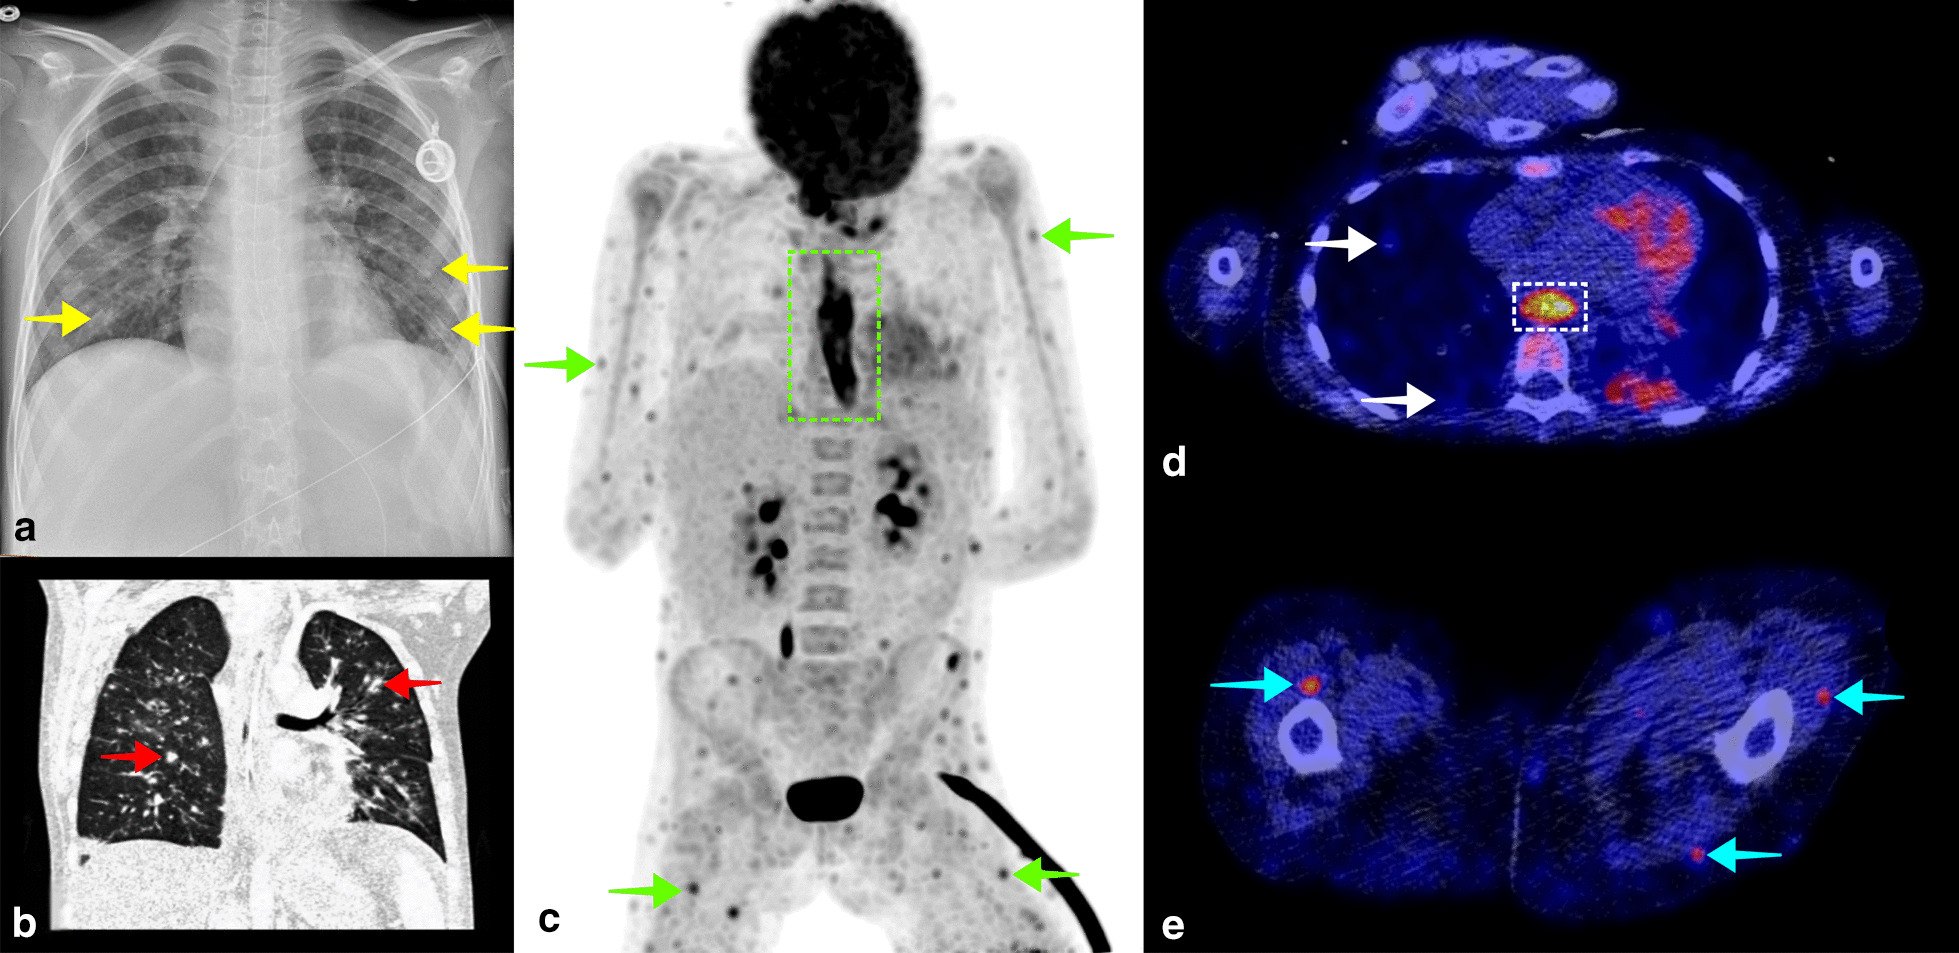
A 10-year-old girl known with acute lymphocytic leukemia was admitted to the hospital because of fatigue and general malaise. During admission, the patient also developed fever, for which blood cultures were taken and cefuroxime was started. Blood cultures were positive for *Candida albicans*. A thoracic X-ray showed small bilateral pulmonary consolidations (a, yellow arrows), and thoracic CT showed multifocal opacities as well (b, red arrows), supporting the diagnosis of pulmonary candidiasis. Voriconazole and caspofungin were started, and a venous access point of the patient was removed because of potential colonization. Despite antifungal treatment, the patient remained febrile, with a CRP level of 61 mg/L and leukocyte count of 23.6 × 10^9^/L. FDG-PET/CT was performed to evaluate other potential foci of infection. Coronal maximum intensity projection FDG-PET showed multiple small subcutaneous and intramuscular FDG avid foci (C, green arrows), and diffuse high FDG uptake in the esophagus (c, dashed green rectangle), suggestive of generalized candidiasis. Small FDG avid pulmonary consolidations were also visible on fused FDG-PET/CT (D, white arrows) as well as high FDG uptake in the esophagus (d, dashed white rectangle), and small subcutaneous and intramuscular FDG avid foci (E, blue arrows). Intensive antifungal therapy was continued, and the patient slowly recovered. The patient was discharged from the hospital 6 weeks after FDG-PET/CT
